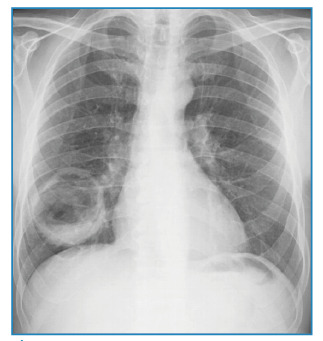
Radiopaque, round-shaped cavitary lesion in the lower lobe of the right lung on chest radiography.
